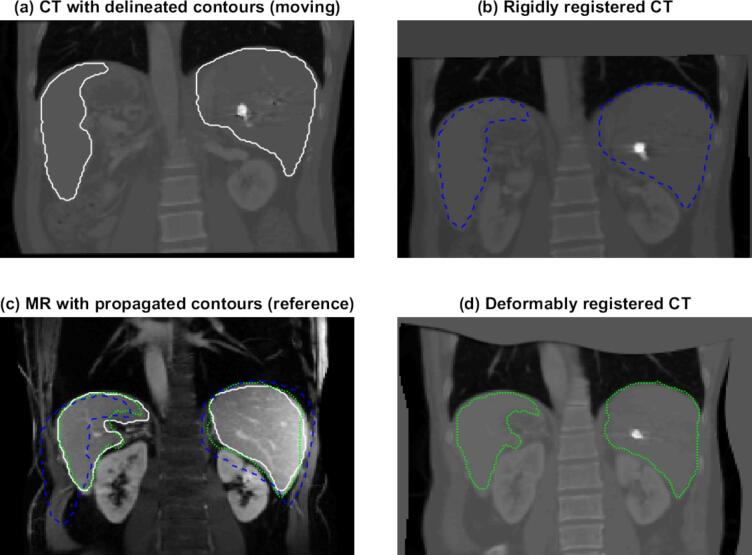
Example of using image registration for contour propagation from a (moving) CT image to a (reference) MR image of the abdomen. Both the result from rigid (blue dashed line, panel (b)) and deformable (green dotted line, panel (d)) image registration are shown. Panel (c) shows these same propagated contours on the MR image, together with the reference contours in white. Images and delineations are adapted from Hering et al. [13]. (For interpretation of the references to colour in this figure legend, the reader is referred to the web version of this article.)
